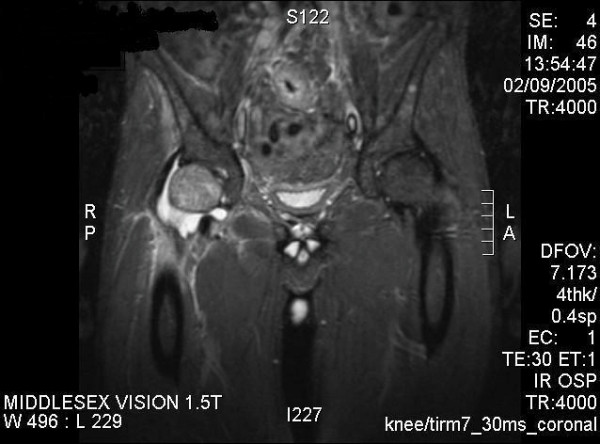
MRI of the pelvis showing effusion with soft tissue swelling and synovial thickening.
